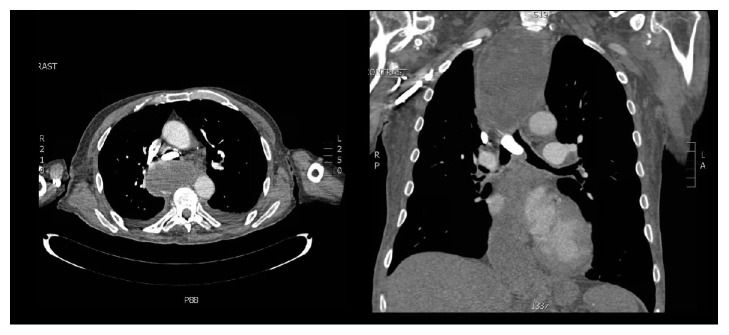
CT chest showing foreign body.
